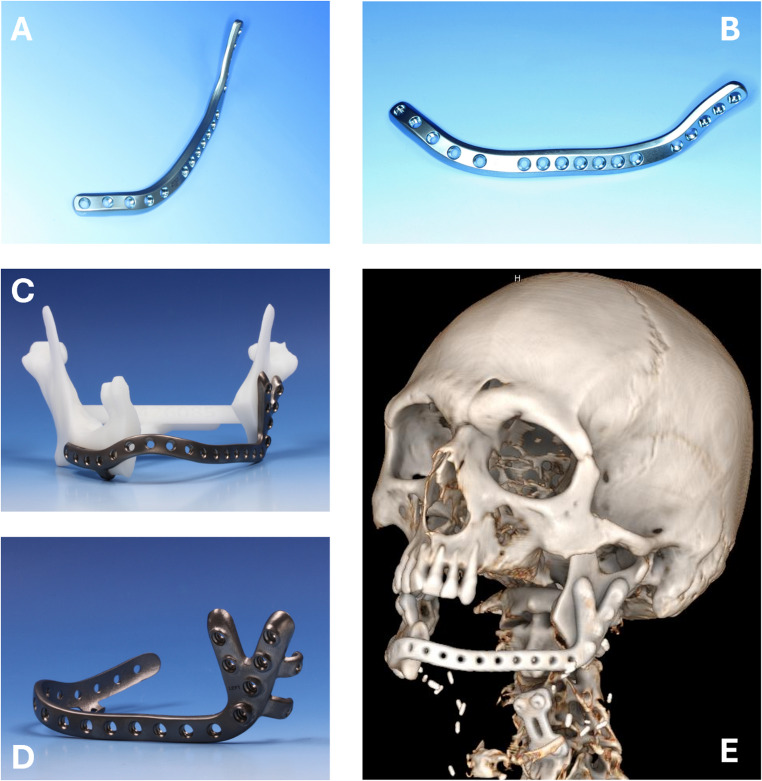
Comparison between first (A and B) and second-generation PSI (C, D, and E). Second-generation PSI offering design benefits for one-fit-only application; frontal (A, C) and lateral view (B, D), and after implantation in virtual reconstruction of a computed tomography (E)
